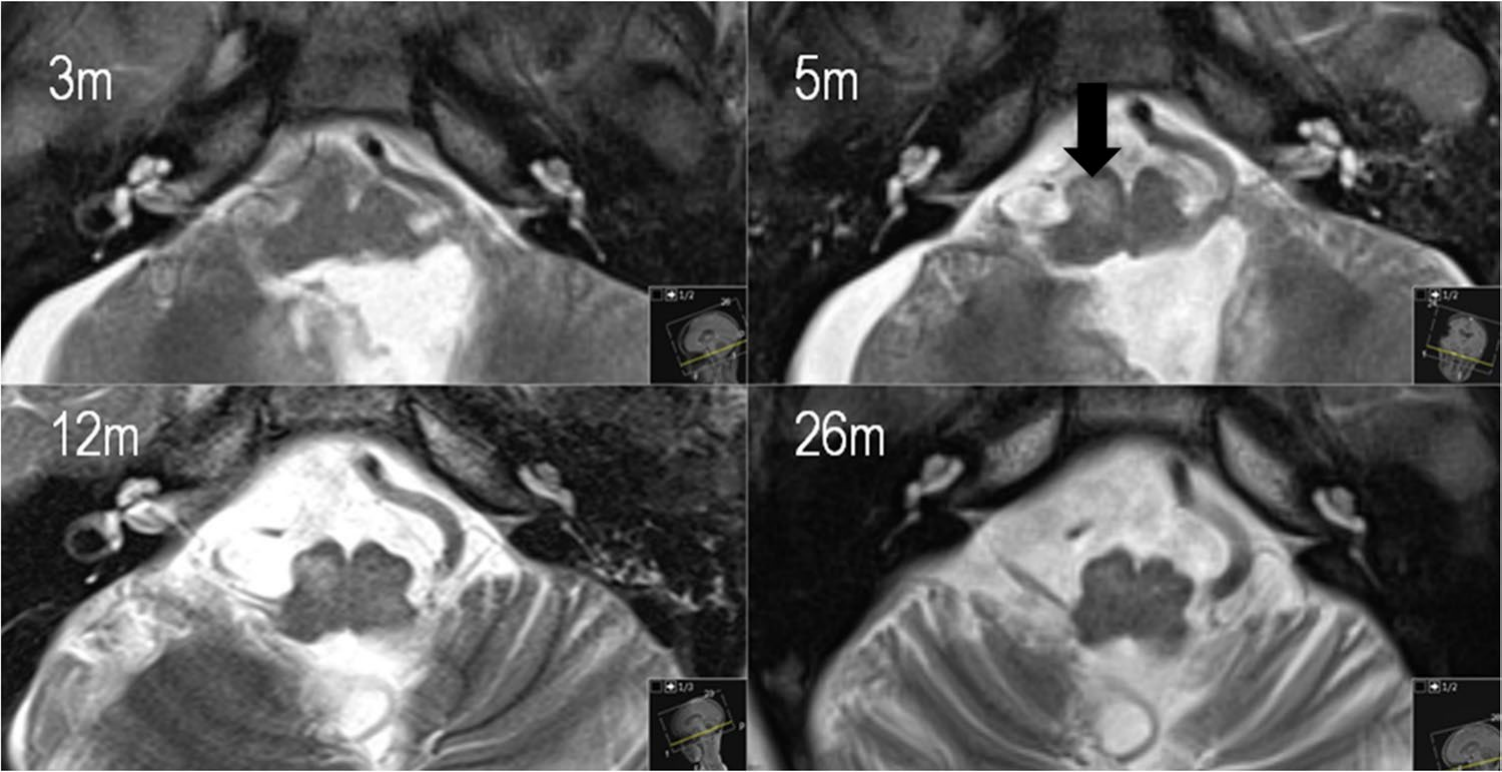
Representative serial postoperative MRI scans of patient CMS_6 (axial T2-weighted sequences, time since tumor resection given in months [m]), with HOD first diagnosed on the MRI scan 5 months after surgery (arrow)
